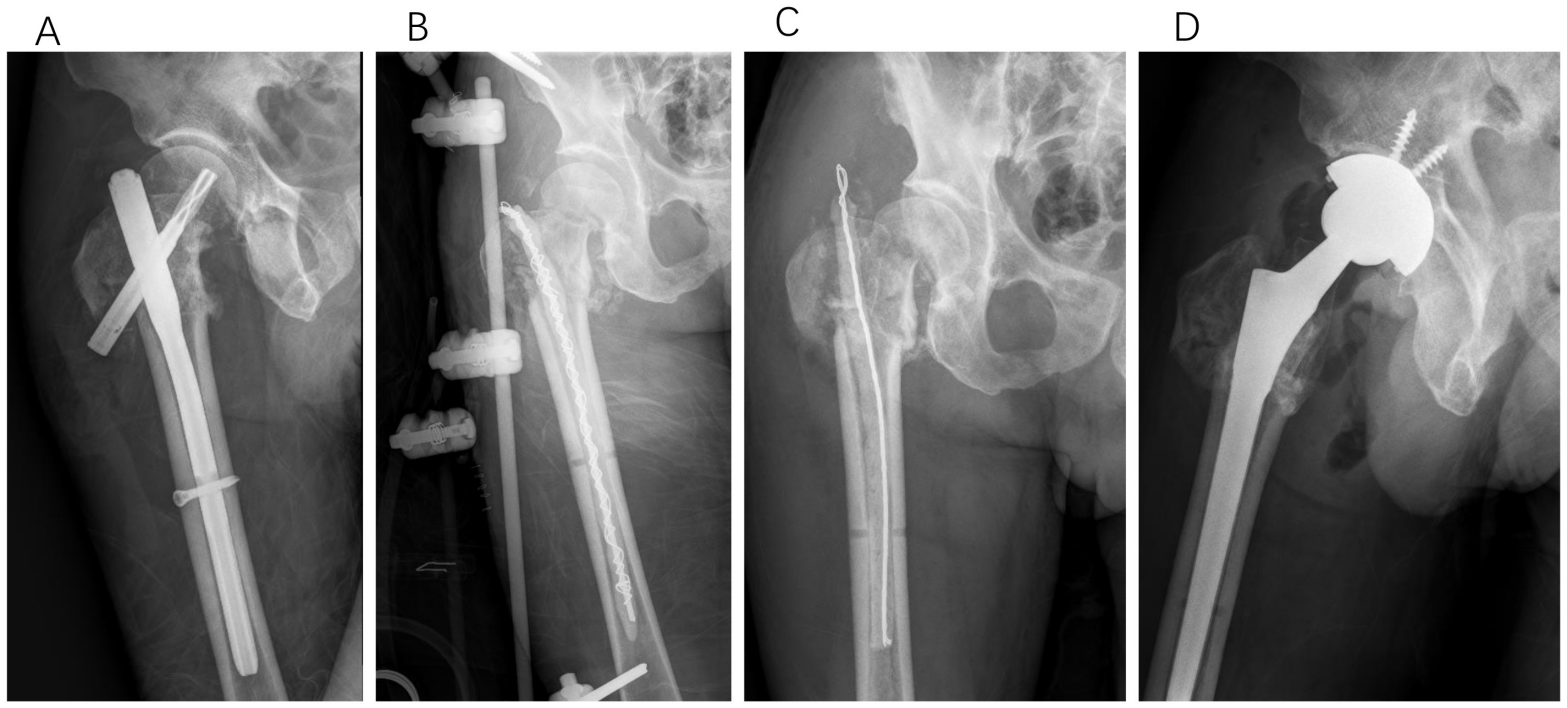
Type IV, a 65-year-old male patient underwent PFNA internal fixation for bilateral intertrochanteric femoral fractures. Post-operatively, the wound on the right side exhibited poor healing, necessitating revision surgery 5 months later. During the subsequent revision surgery, we performed extensive debridement, filled the medullary cavity with an antibiotic-impregnated cement rod, and provided temporary stabilization using an external fixator. After 2 months, a second debridement was conducted, and the antibiotic-impregnated cement rod was replaced. Following 4.5 months of treatment, the infection was successfully controlled. Due to the limited residual bone mass of the femoral head and the high risk of necrosis, total hip arthroplasty was ultimately performed. (A) Anteroposterior X-rays obtained 5 months after initial PFNA fixation. (B) Anteroposterior X-rays acquired immediately following the first revision surgery. (C) Anteroposterior X-rays acquired immediately following the second revision surgery. (D) Anteroposterior X-rays acquired immediately following the final revision surgery.
